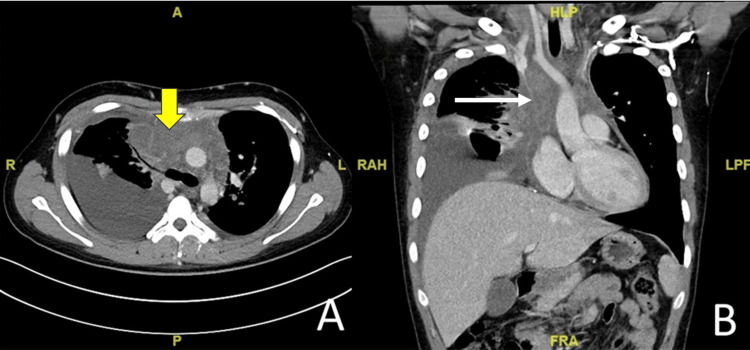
Post-intravenous contrast medium computed tomography of the patient, in axial (A) and coronal (B) planes, demonstrates a soft tissue mass within the mediastinum (yellow arrow), with resultant significant narrowing of the superior vena cava (white arrow).
